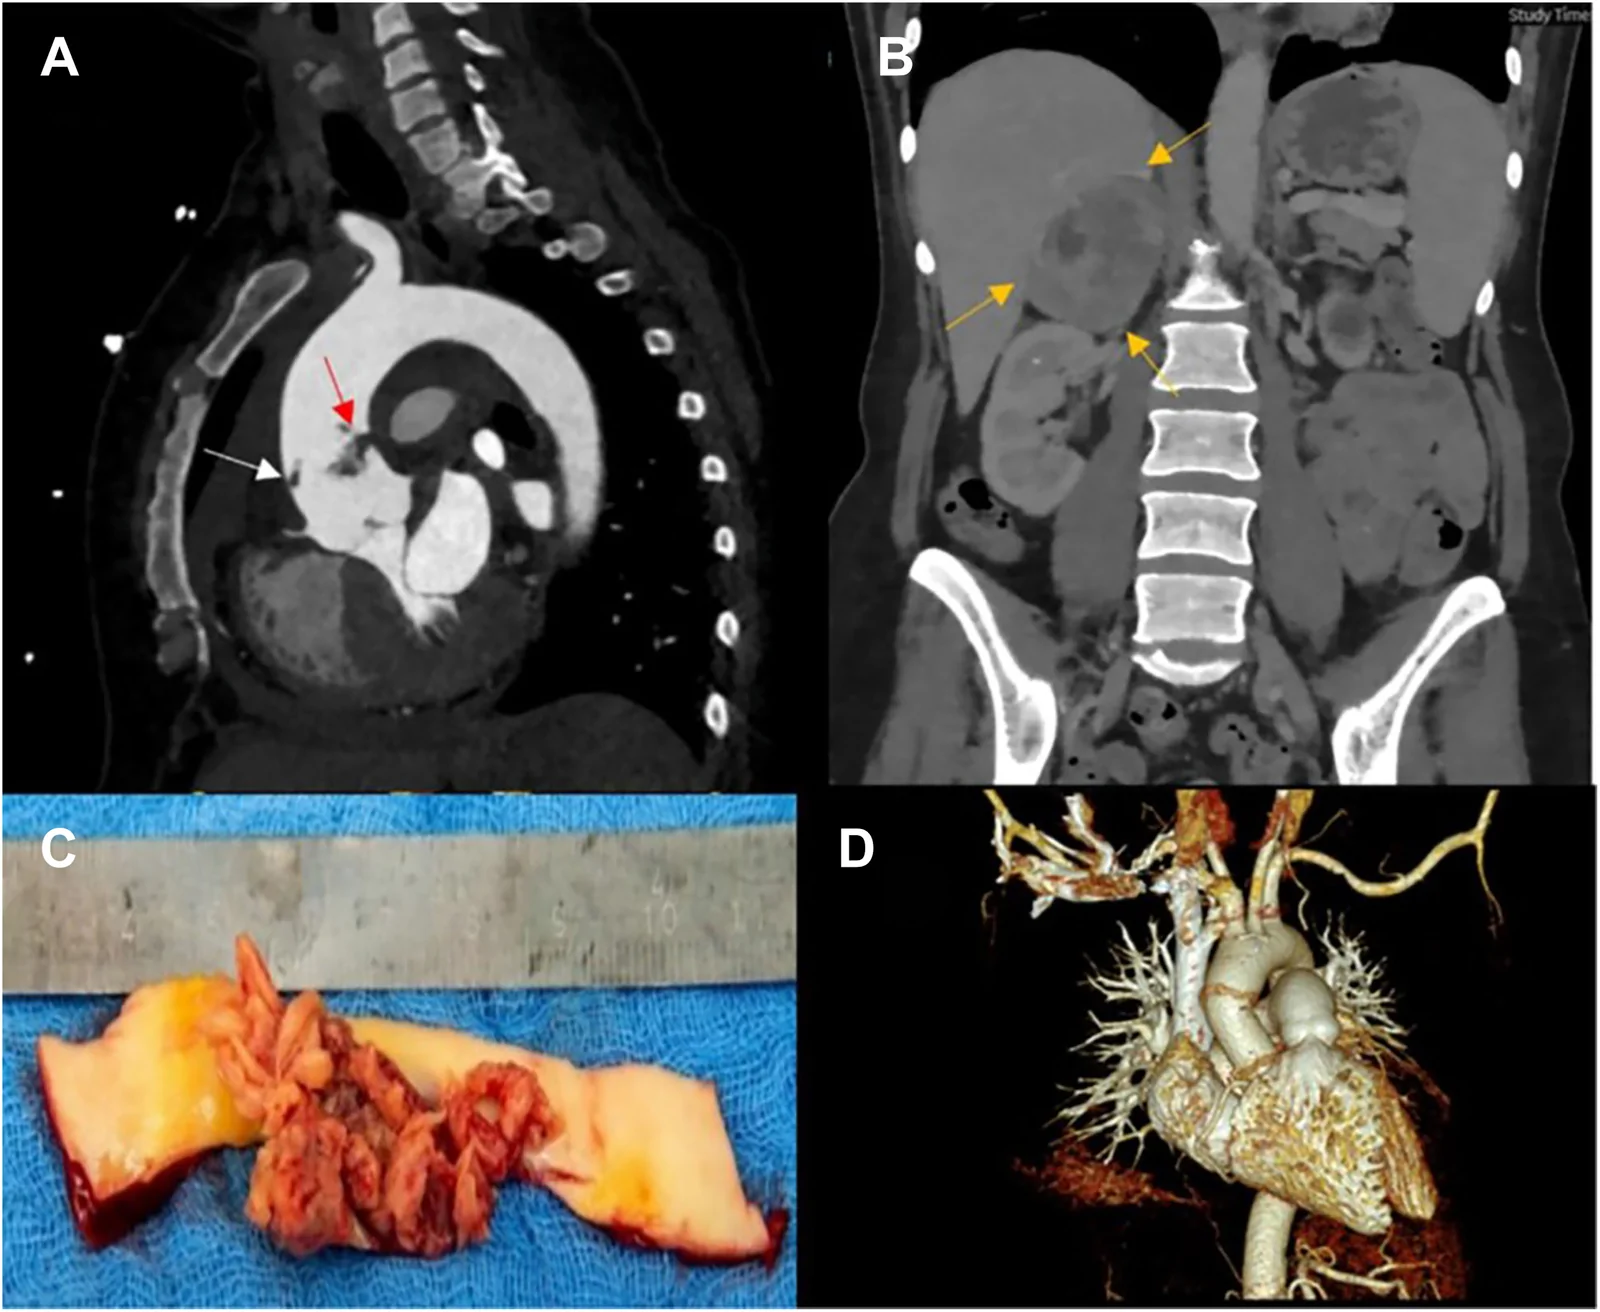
(A) Computed tomography shows aortic wall injury (white arrow) and an intramural thrombus (red arrow). (B) Incidental right adrenal mass (arrows). (C) Gross pathology of a part of the injured wall of the ascending aorta, including a mural thrombus. (D) Three-dimensional computed tomography reconstruction of the thoracic aorta shows the replacement of the ascending aorta.
